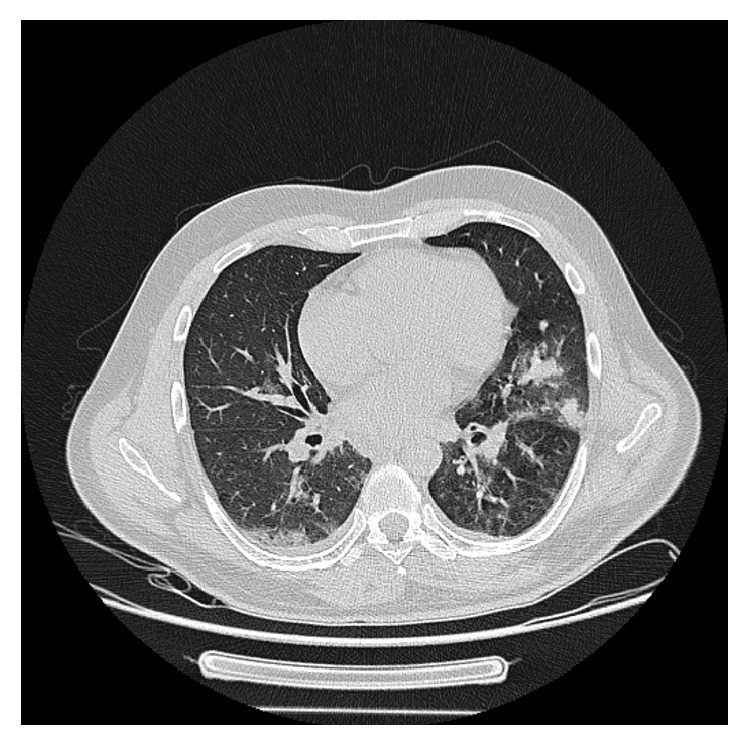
Computed tomography scan of the chest demonstrating diffuse bilateral opacities, mainly at the left side.
